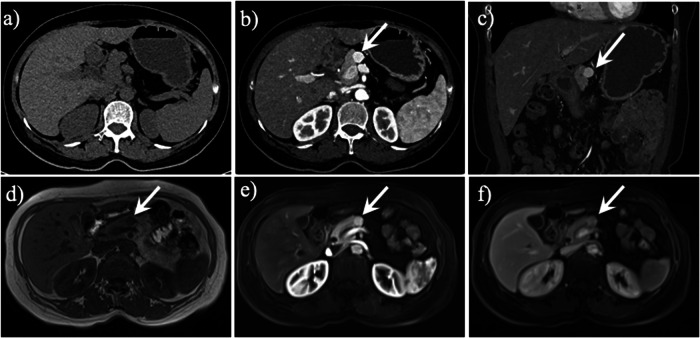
A 62-year-old woman with intra-pancreatic fat deposition coexisted with a pancreatic neuroendocrine tumor. Axial unenhanced and contrast-enhanced computed tomography (CT) images (a, b) and coronal contrast-enhanced CT image (c) showed only the head of the pancreas (arrow), while the pancreatic body-tail portion showed fat density. There was an obvious homogeneous hypervascular nodule in the neck of the pancreas (arrow), which was confirmed as a neuroendocrine tumor by postoperative pathology. Axial T1-weighted non-contrast (d), arterial phase (e), and venous phase (f) images demonstrated ‌fat-equivalent signals‌ in the pancreatic body and tail‌ region. A ‌nodule in the pancreatic neck‌ exhibits ‌mild hypointensity‌ on non-contrast T1WI ‌(arrow) ‌, with ‌marked arterial phase enhancement ‌(arrow) and ‌venous phase enhancement intensity‌ comparable to that of the pancreatic head parenchyma (arrow)
